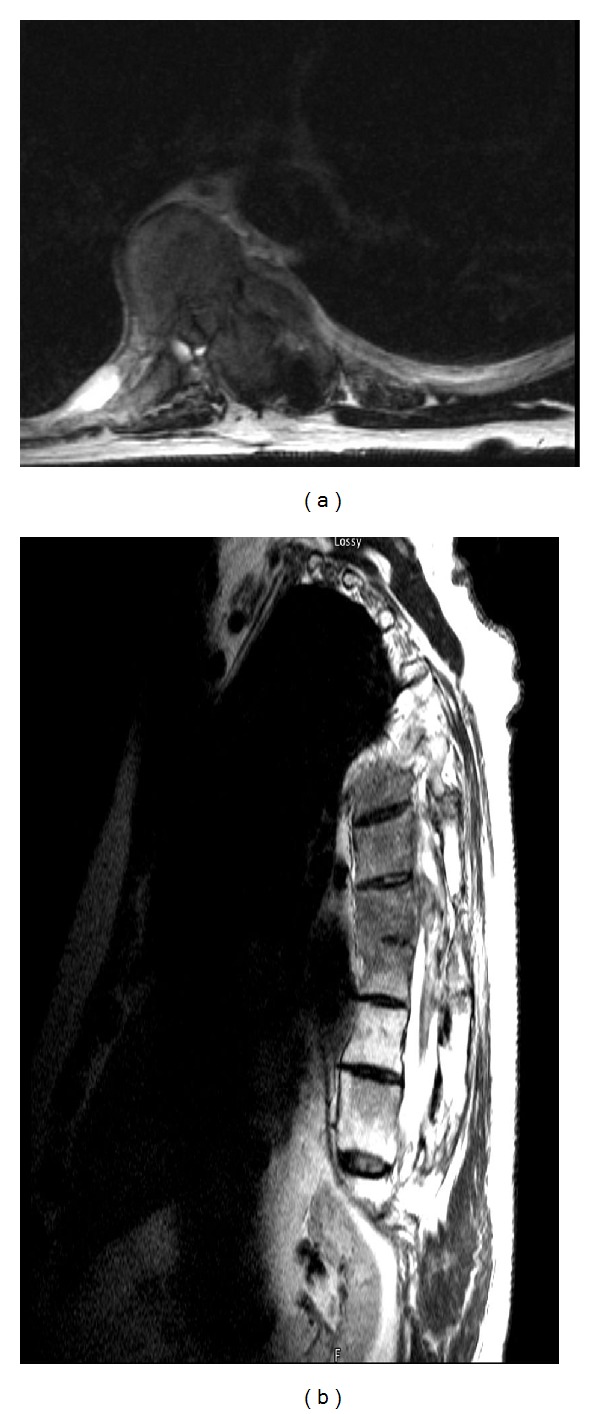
A year after his second resection. Axial and sagittal T2 MRI images of Thoracic spine show anterior lateral tumor recurrence with spinal cord compression to the left. Complete CSF block at T8-9 was noted.
